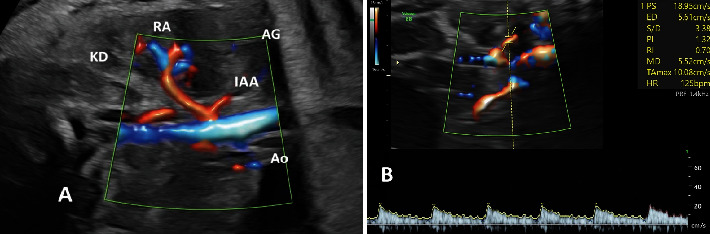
(A) Doppler color flow of IAA in oblique-coronal plane. (B) The IAA Doppler pulse waves and indices. Abbreviations: AG, adrenal gland; Ao, aorta; IAA, inferior adrenal artry; KD, kidney; RA, renal artery.
